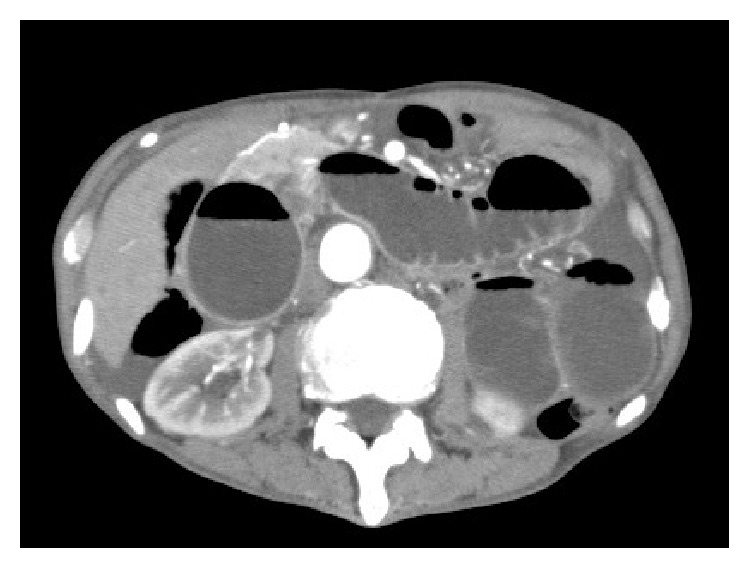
Abdominal computed tomography scan revealed dilation of the duodenum and small intestine in the left upper quadrant.
